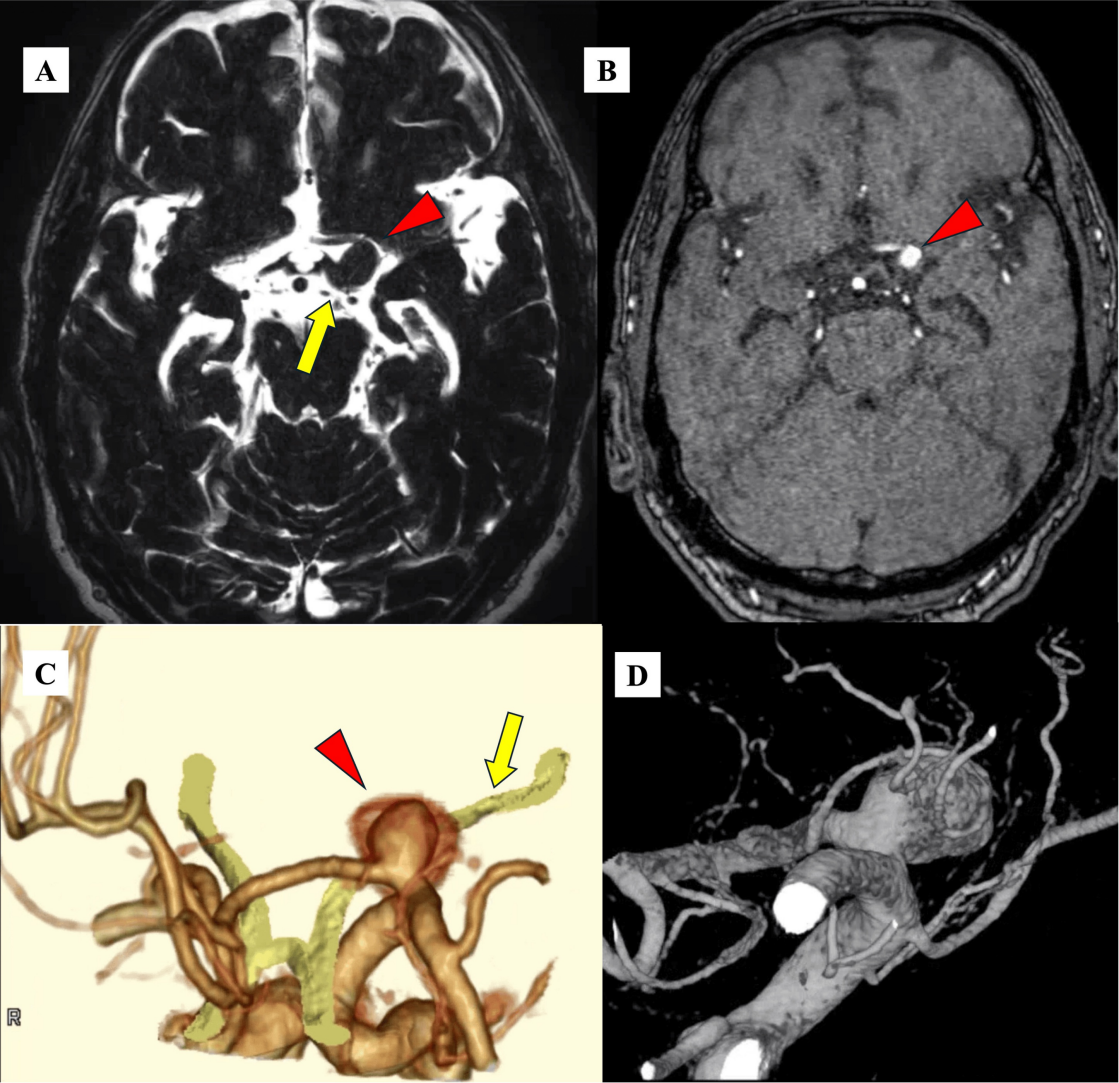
Preoperative images of the A1 aneurysm A, B: magnetic resonance (MR) cisternography and MR angiography revealing a thrombosed A1 aneurysm (red arrowhead) compressing the left optic tract (yellow arrow) from the anterior lateral side. C: 3D reconstruction of computed tomography angiography showing the aneurysm (red arrowhead) compressing the left optic tract (yellow arrow) from the anterior lateral side. D: 3D reconstruction of the left internal carotid angiography showing the A1 aneurysm projecting posteriorly and no perforators arising from the neck of the aneurysm.
